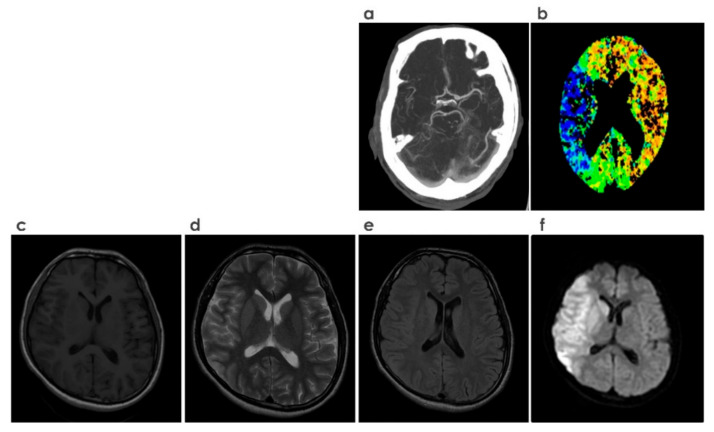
Various neuroimaging modalities. (a) CT Angiography, (b) CT Perfusion, (c) T1–weighted imaging, (d) T2–weighted imaging, (e) FLAIR (fluid attenuated inversion recovery), (f) DWI (diffusion weighted imaging).
